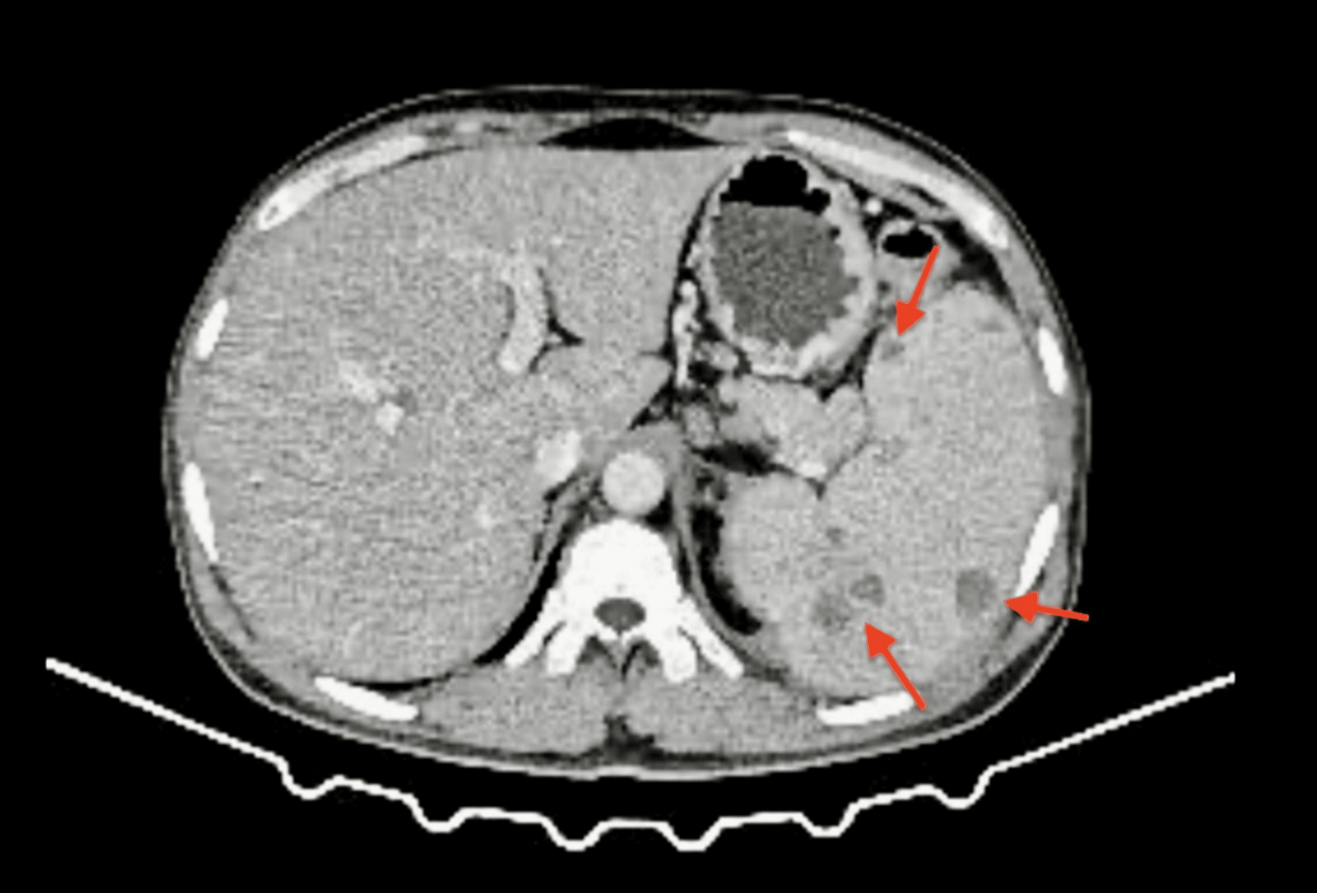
Axial section of the CECT abdomen showing multiple hypodense lesions in the spleen (arrows) CECT: contrast-enhanced computed tomography
